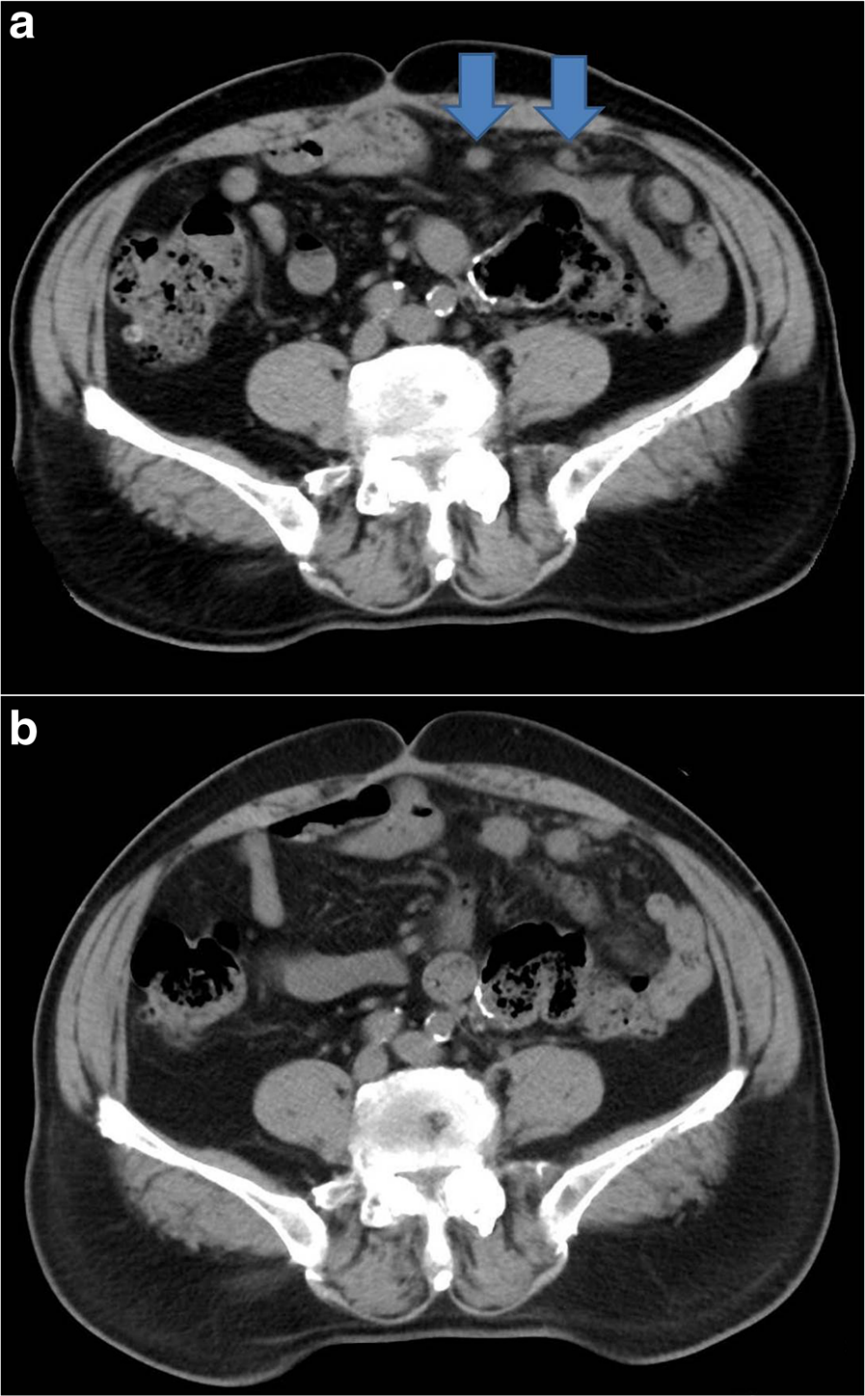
CT imaging. a Soft-tissue density masses arose in the left lower abdominal quadrant 9 months after radical surgery, as indicated by the *arrows*. b The masses grew in size during the ensuing 3 months
